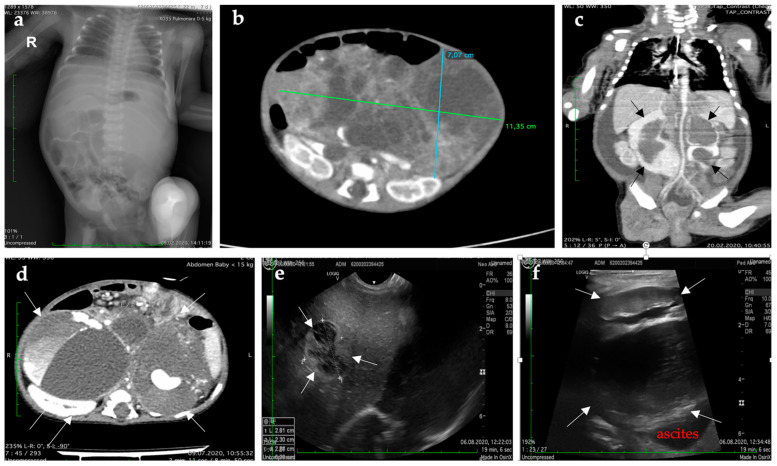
Postnatal imagistic findings. (a) Initial preoperative radiological evaluation showing ascension of the diaphragm due to pancreatic tumor mass. (b) Preoperative CT scan reveals a large mass in the fetal pancreas. (c) Postoperative CT scan showed residual tumor. (d) CT scan reveals multiple hepatic tumors with cystic components (arrows). (e) Ultrasonography showed liver relatively homogeneous with a nodular image of 23 mm with mixed appearance, predominantly transonic in segment VII. (f) Ultrasonography revealed multiple hepatic tumors with cystic components (arrows).
